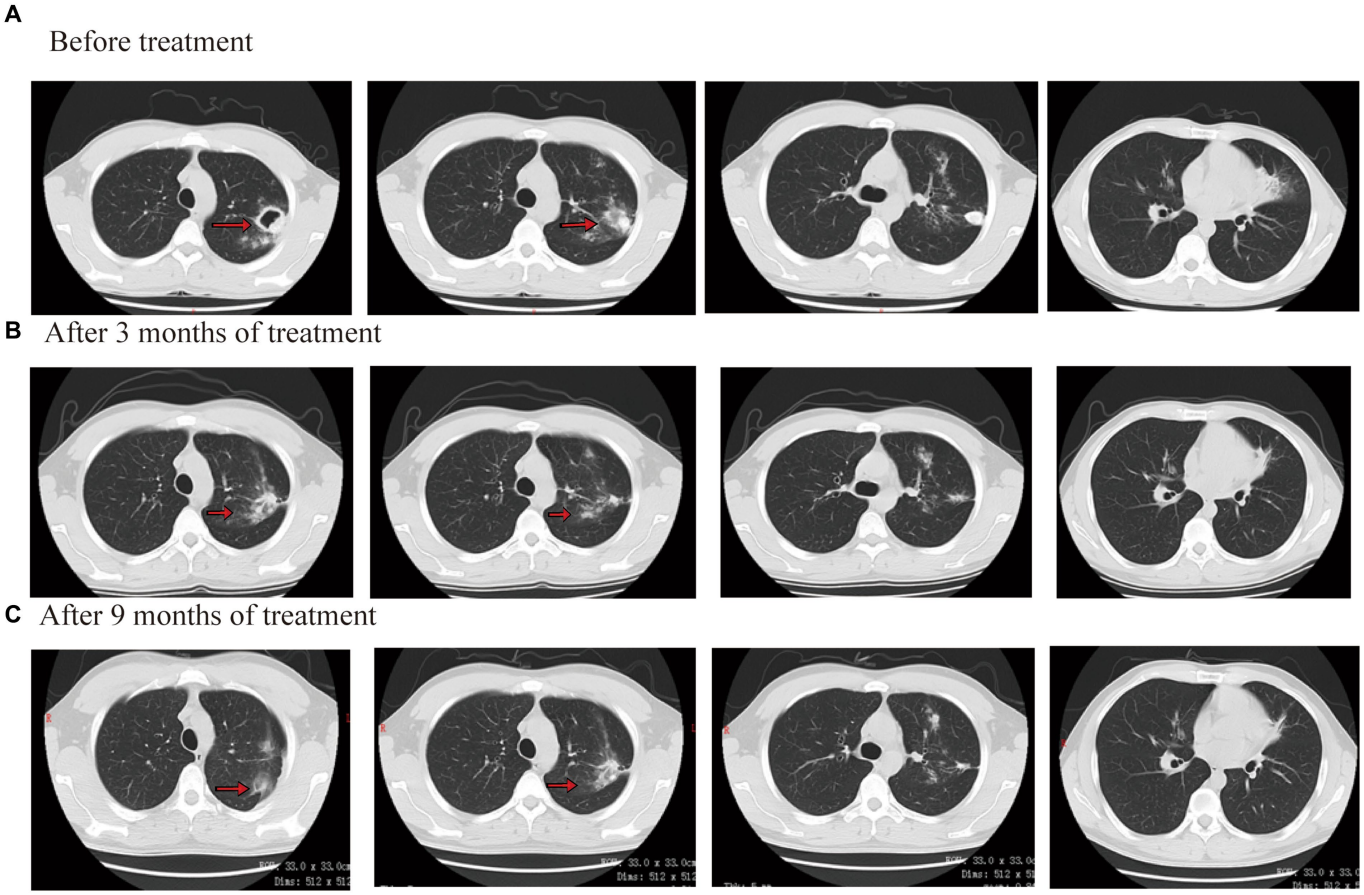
Chest CT scan images before and after treatment for case 3.
